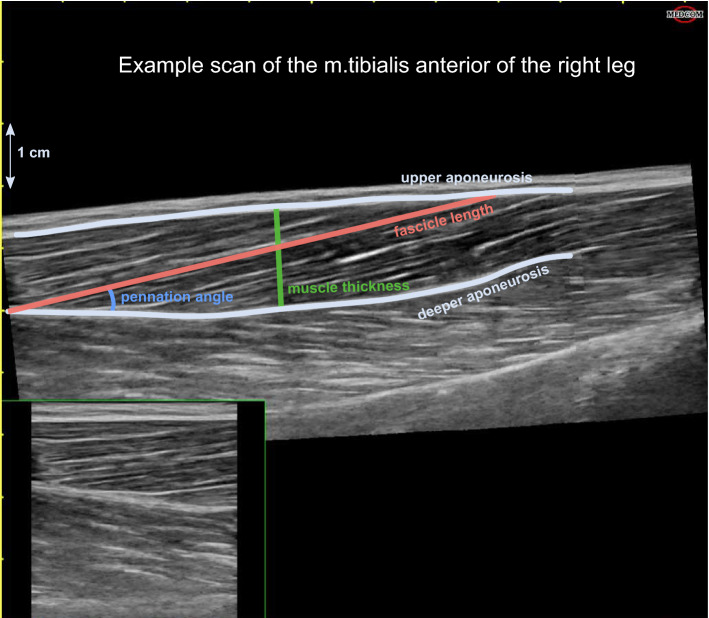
Representative ultrasound scan of the m. tibialis anterior at rest for a younger participant. The muscle architecture is color-annotated as follows, light blue/grey: upper and deeper aponeurosis; red: fascicle length; blue: pennation angle; green: muscle thickness
